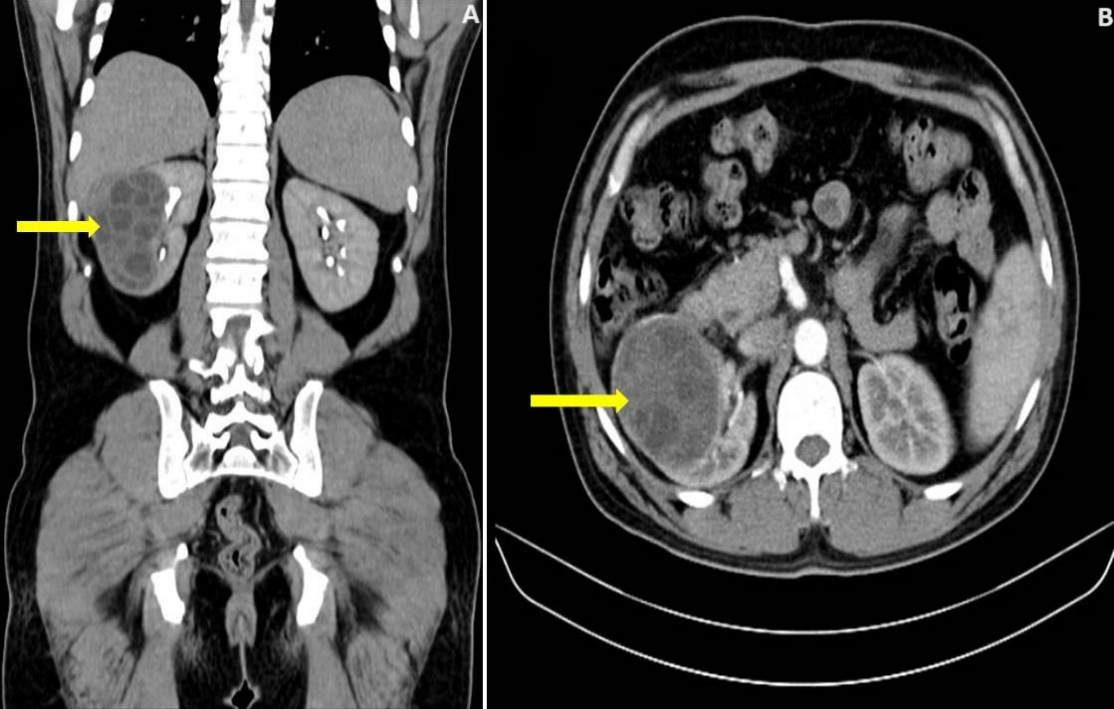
(A,B) CT scan of the abdomen with a yellow arrow showing multiloculated cystic lesion with calcifications in the right kidney
